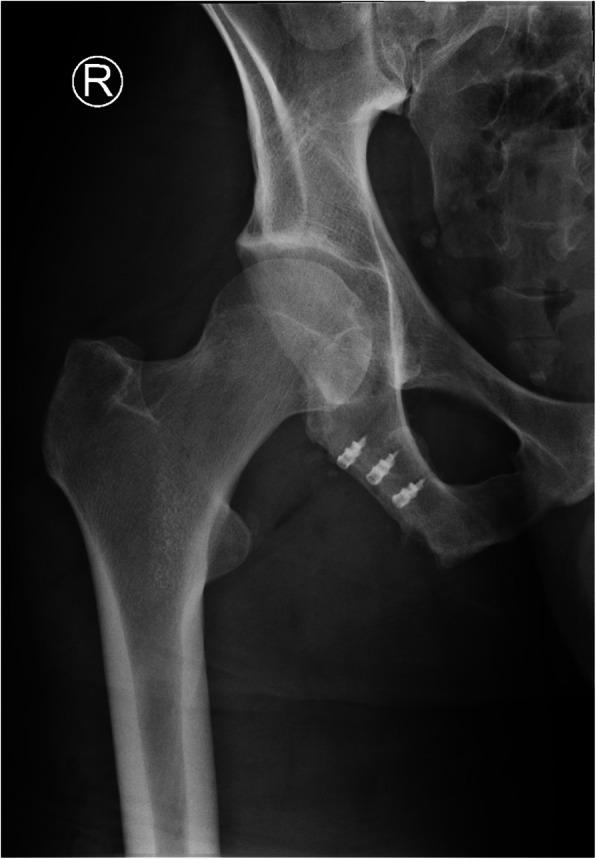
Postoperative radiograph showed correct position of three suture anchors and total excision of the nonunion fragment
